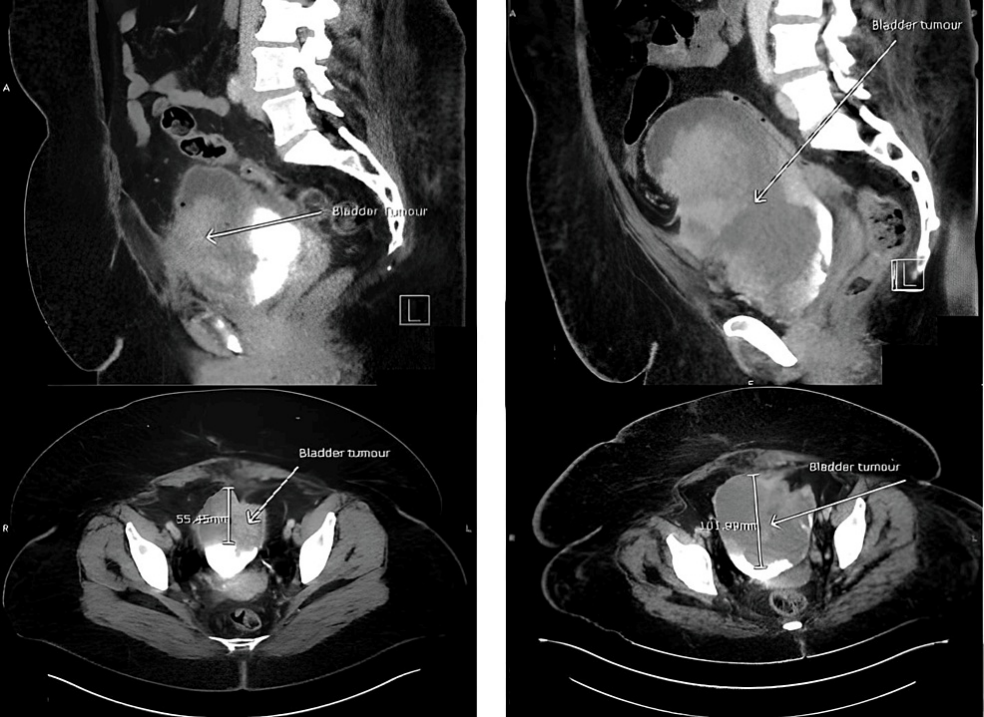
Comparative CT-Urography images showing progression of bladder mass. Cross-sectional images (sagittal and axial) - (Left) at presentation vs (Right) eight weeks later
